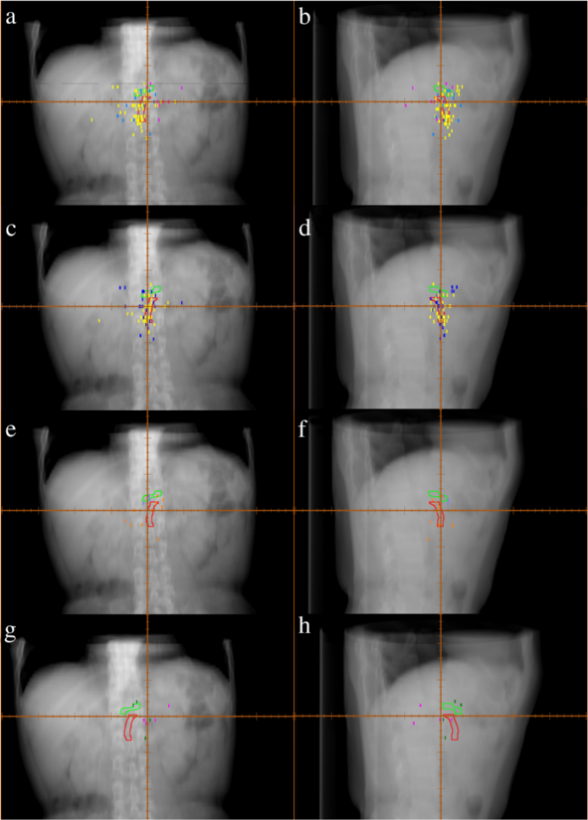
Digitally reconstructed radiograph of local recurrences. a Anterior-posterior and b lateral views of local recurrence plots with respect to the superior mesenteric artery (*red*) and celiac artery (*green*) for postoperative patients with pancreatic head(*yellow*), body(*blue*), and tail (*peach*) cancer. c Anterior-posterior and d lateral views of local recurrence plots with respect to the superior mesenteric artery (*red*) and celiac artery (*green*) for postoperative patients with chemotherapy alone (*yellow*) or no adjuvant therapy (*blue*) in pancreatic head cancer. e Anterior-posterior and f lateral views of local recurrence plots with respect to the superior mesenteric artery (*red*) and celiac artery (*green*) for postoperative patients with chemotherapy alone (*orange*) or no adjuvant therapy (*lavender*) in pancreatic head cancer. g Anterior-posterior and h lateral views of local recurrence plots with respect to the superior mesenteric artery (*red*) and celiac artery (*green*) for postoperative patients with chemotherapy alone (*peach*) or no adjuvant therapy (*dark green*) in pancreatic head cancer
